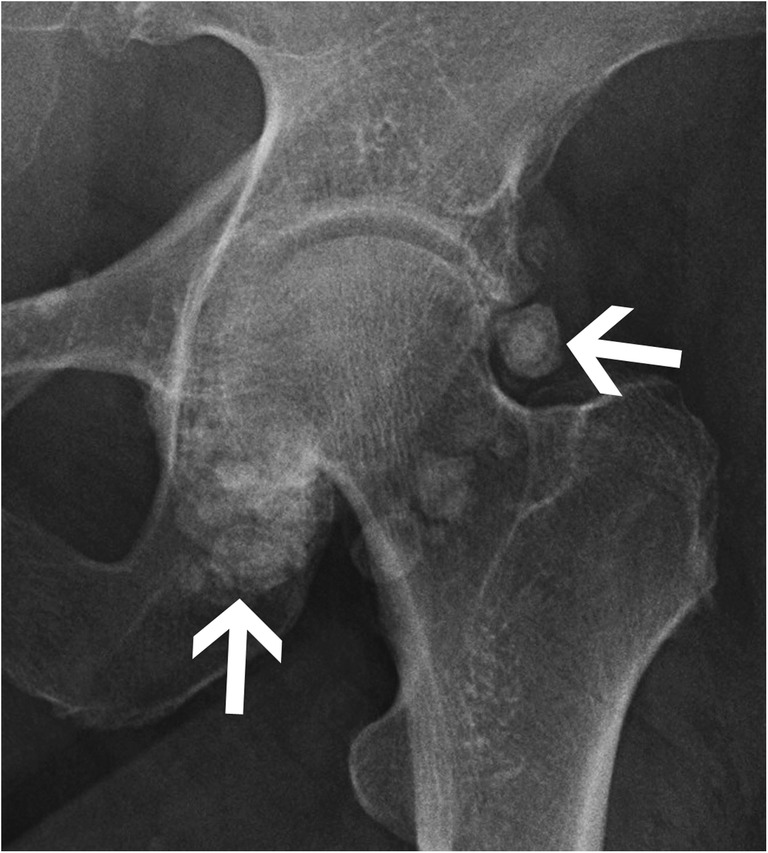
Synovial osteochondromatosis. Anteroposterior radiograph of the left hip shows multiple intra-articular osteocartilaginous bodies (arrows) with ring-like calcifications centred on the coxofemoral joint
